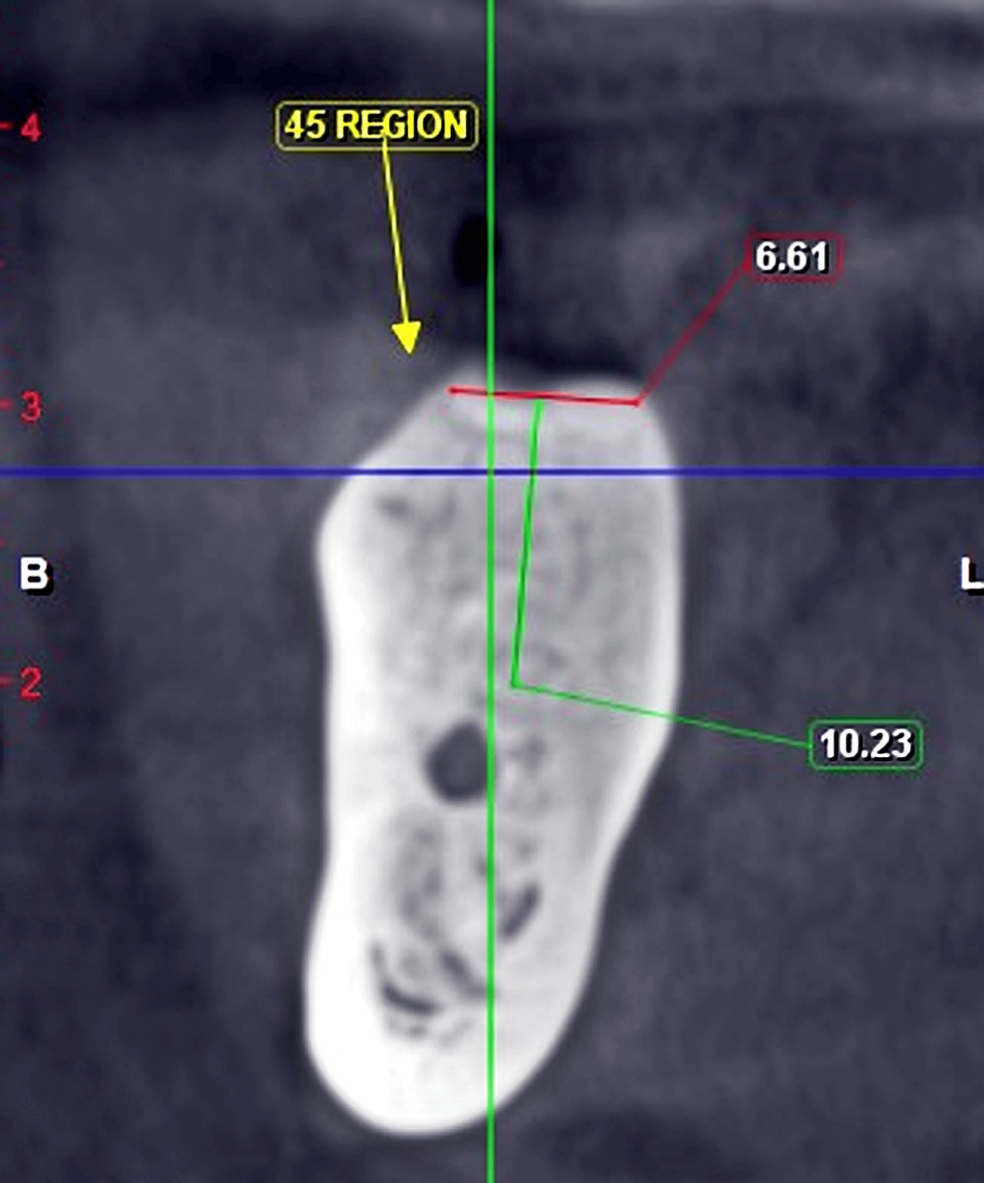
CBCT image a (sagittal section, 2 mm slice view) The cone beam computed tomography (CBCT) image shows available bone height and width in the 45 region.
